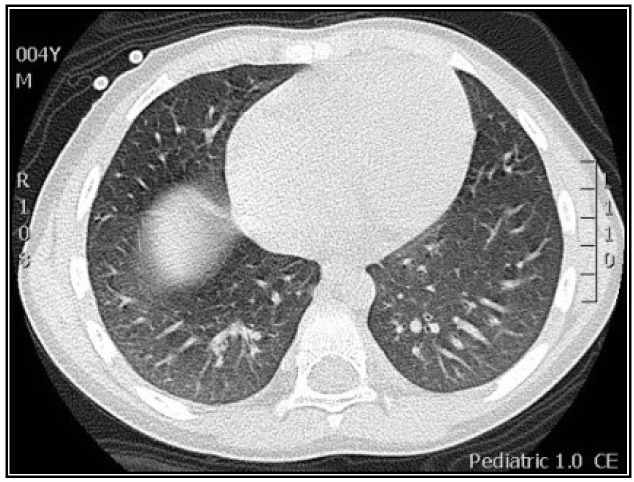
Ground-glass pattern in CMV lung infection detected with high resolution CT scan (HRCT). Four year old boy with hypoxemia and diffuse rales/wheezing after bone marrow transplant. Plain CXR revealed non-specific mild increased lung markings. HRCT identified subtle ground-glass pattern in both bases. CMV diagnosis was confirmed by (+) PCR in bronchoalveolar lavage.
